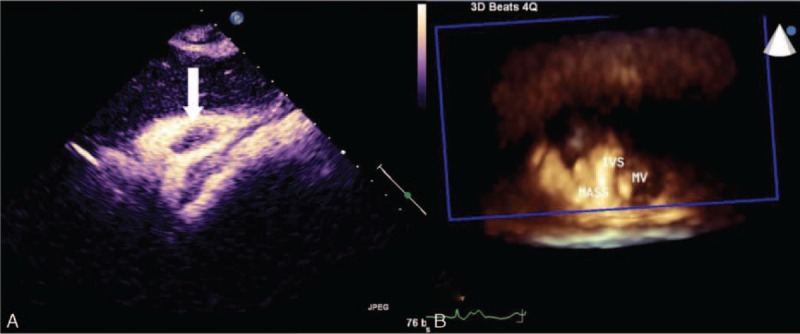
Contrast enhancement ultrasound findings show few contrast agent enhancements in the tumor structure (white arrow; A); real-time 3D full-volume bird's eye view findings of the mass adherent to the IVS and attached to the RV wall (B). IVS = interventricular septum; MV = bicuspid valve; RV = right ventricle.
